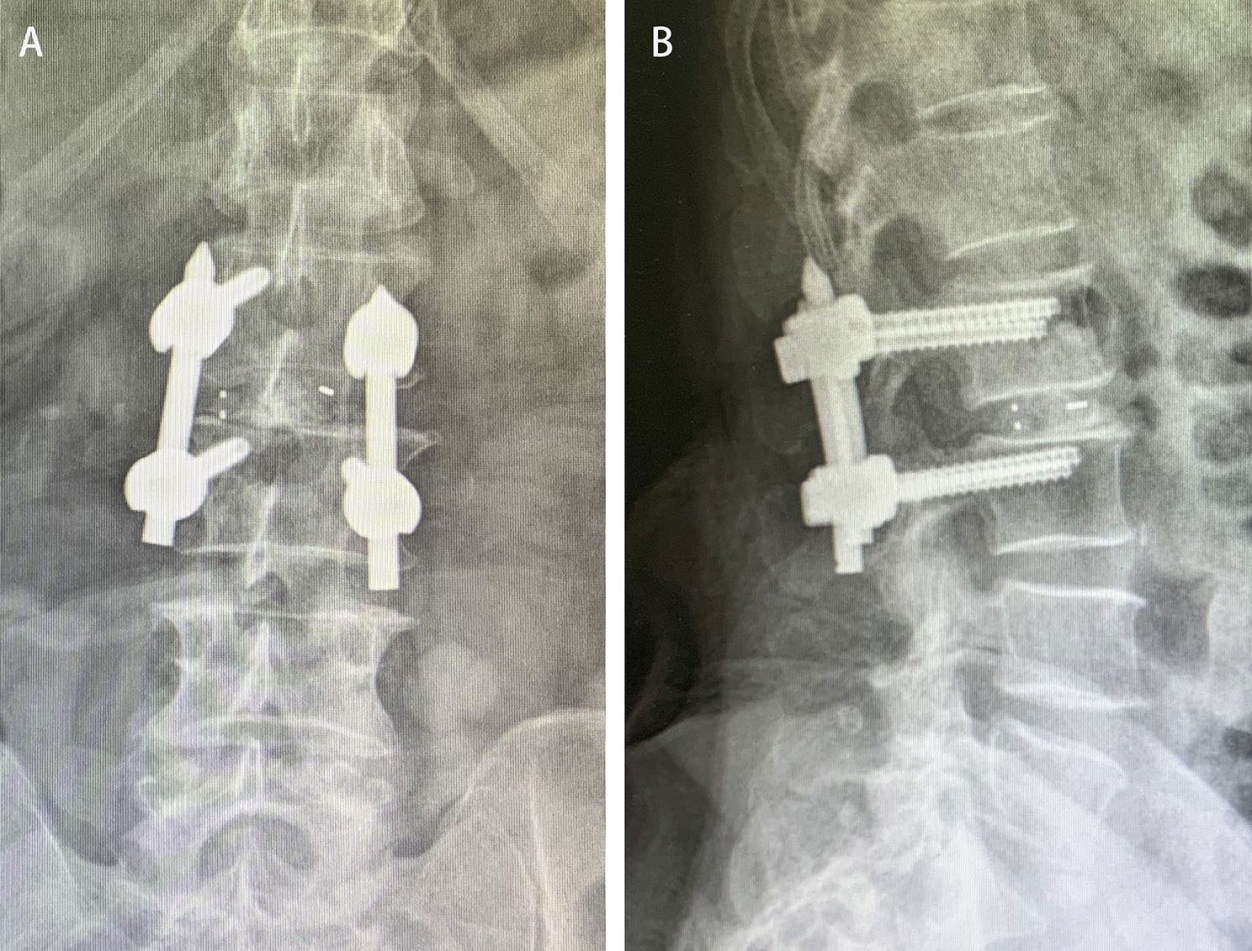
Postsurgical anteroposterior and lateral radiographs showing satisfactory positions of the fusion cage and internal fixators. (A) Anteroposterior image. (B) Lateral image
